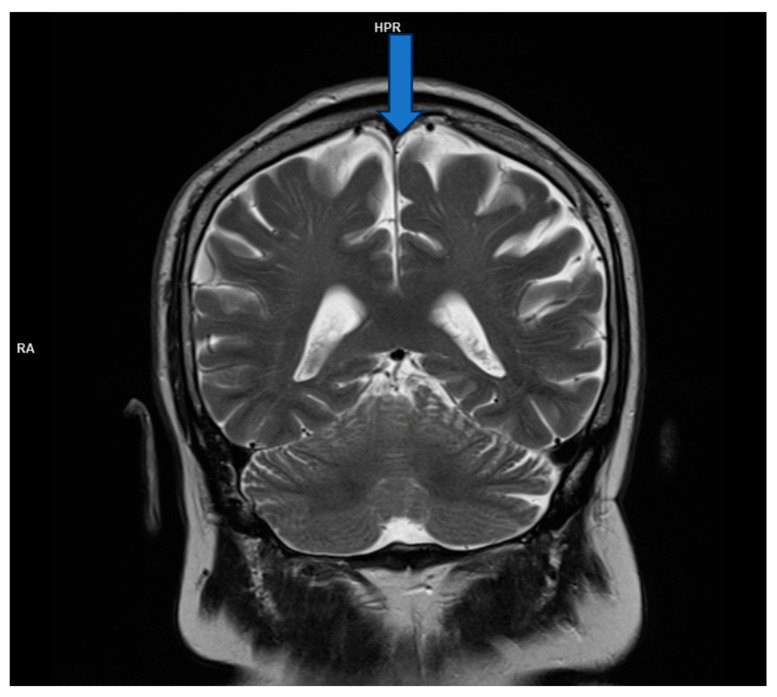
MRI; T2 coronal section- cortical atrophic changes.
